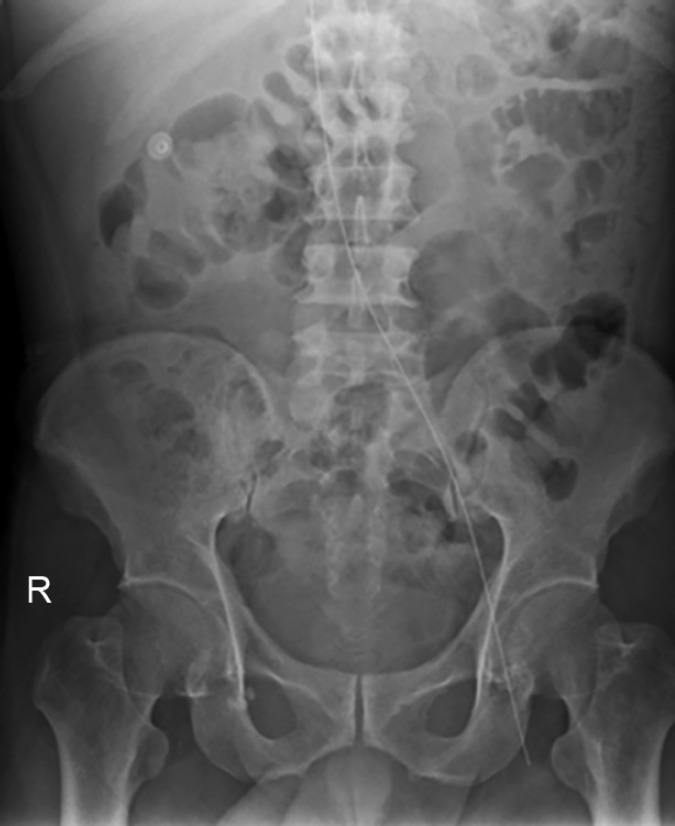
X-ray abdomen and pelvis showing the migrated guidewire in situ with distal end lying in femoral vein
